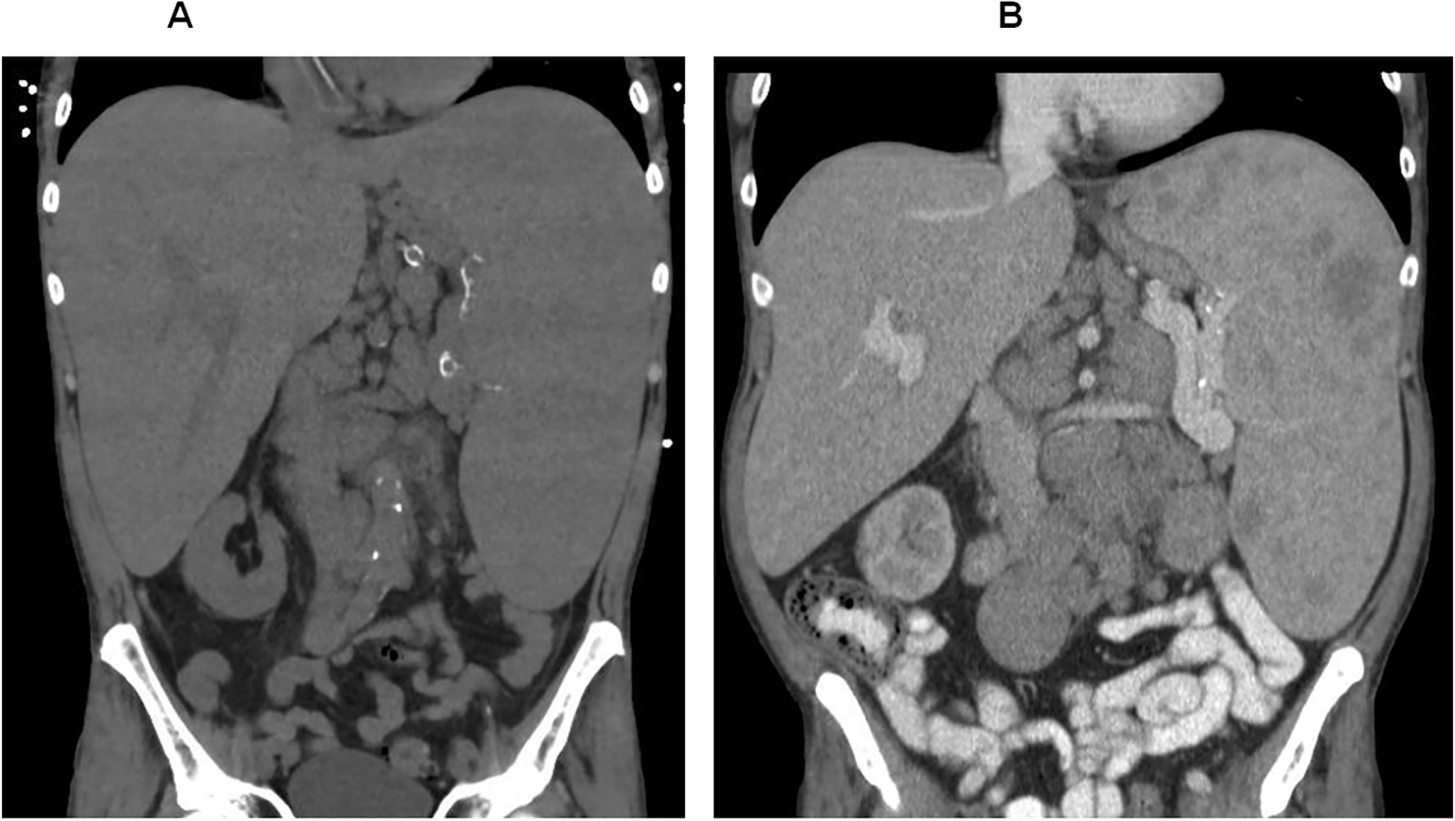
(A) Abdominal computerised tomography (CT) scanning conducted in May 2012. Before treatment with liposomal daunorubicin. (B) Abdominal computerised tomography (CT) scanning conducted in July 2013. After treatment with liposomal daunorubicin, demonstrating no decrease in the patient’s hepato-splenomegaly.
